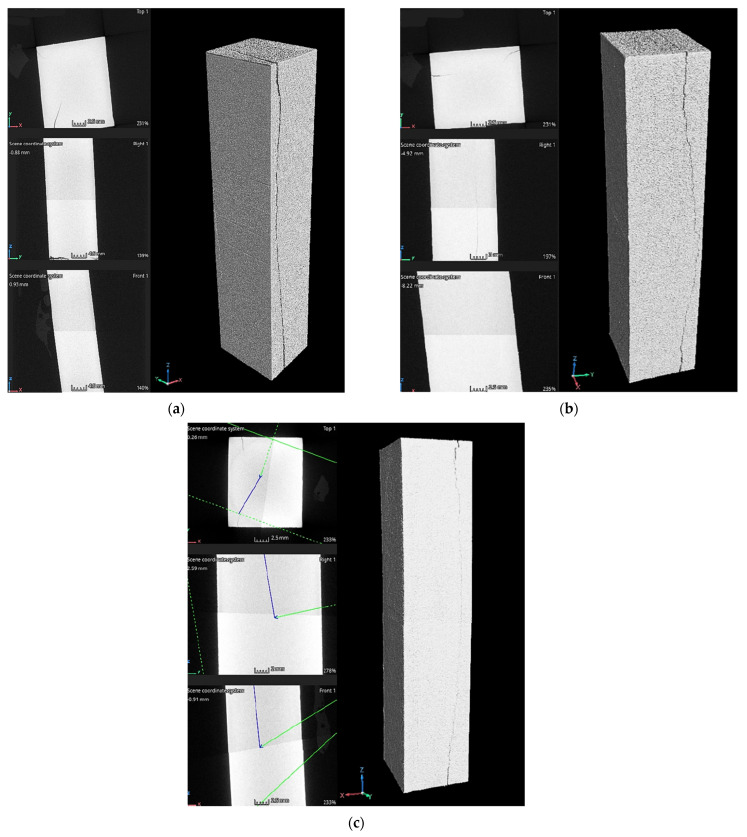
CT scans of the LPBF-printed samples after hot isostatic pressing: (a) 2XY, (b) 3XY, and (c) 4XY.
